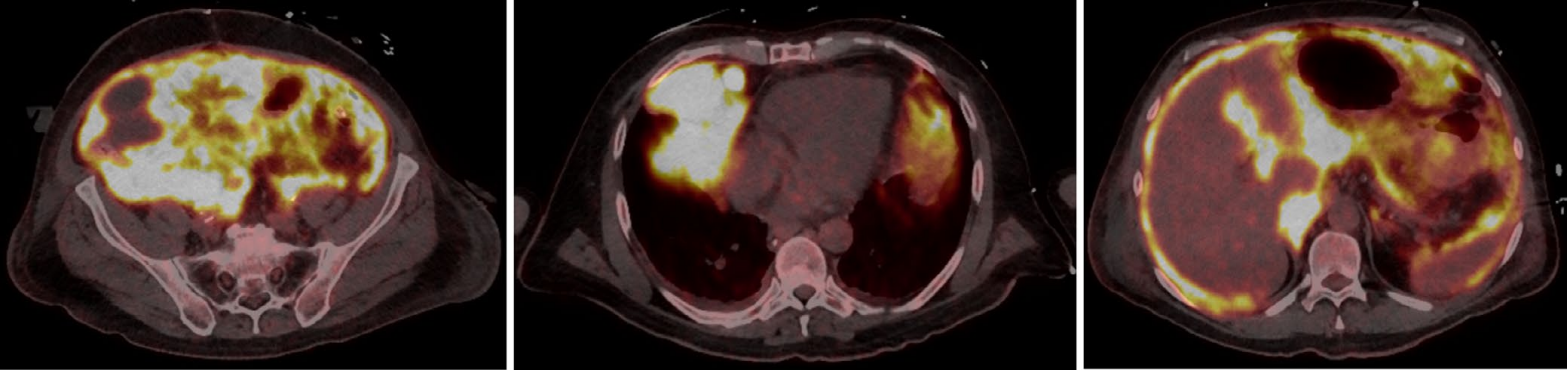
Left: Transversal image from patient's abdominal positron emission tomography–computed tomography (PET‐CT) post tumoral resection revealing diffuse peritoneal hypermetabolism with difficult differentiation between an infectious versus an oncological process. Middle: Transversal image from patients thoracic PET‐CT revealing supradiafragmatic invasion of the lymphoma with a mass and a nodule located in the right cardiophrenic recess with diffuse bilateral pleural nodular thickening. Right: Transversal image from patient's abdominal PET‐CT showing retroperitoneal adenopathies.
